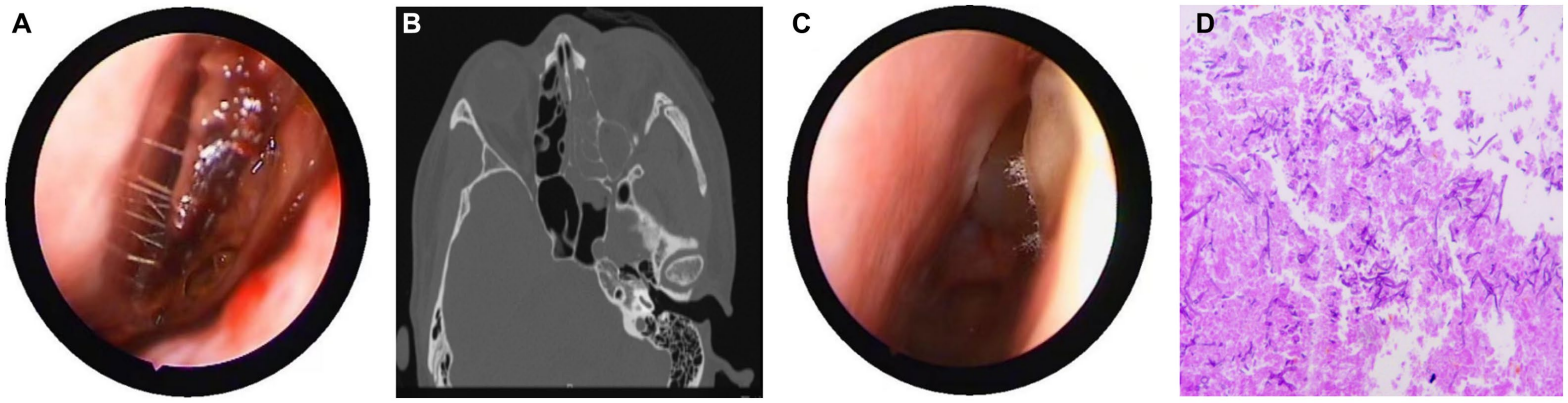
Nasal endoscopy, sinus CT, and pathological results. (A) Endoscopic examination showing pus in the left middle meatus and blood scab on the surface of the middle turbinate. (B) CT showed left ethmoid and frontal sinusitis, with thickening and swelling of the left medial rectus and inferior rectus muscles, and swelling of the soft tissues around the left orbit and cheek. (C) During the intraoperative nasal endoscopy, white hyphae were found in the left nasal cavity. (D) Postoperative pathology: a large number of fungal hyphae and spores were seen in the nasal and sinus mucosa.
